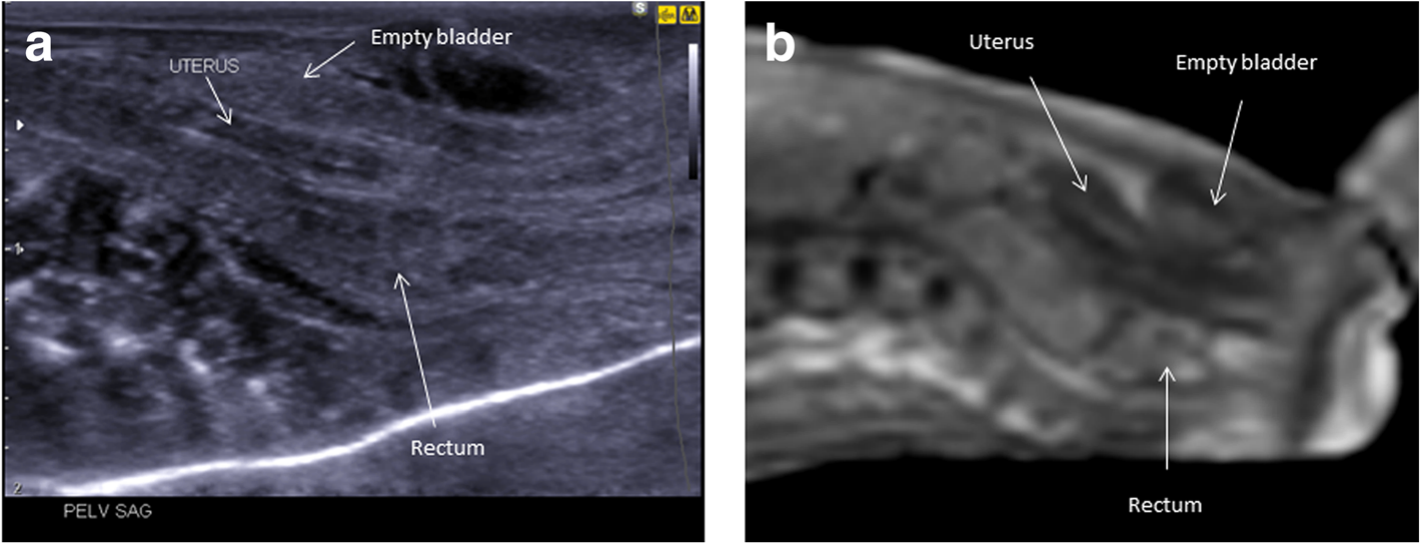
These images demonstrate normal appearances of the pelvic structures in a female 18-week gestational-aged foetus. Sagittal postmortem ultrasound of the pelvis (a) and corresponding postmortem T2-weighted MRI at 1.5 T (b) have been obtained 12 days after death. The tubular appearances of a normal pre-pubertal uterus, rectum and urinary bladder are all well seen and labelled in these images. Using a high-frequency linear probe, the pelvic anatomy can be better demonstrated on ultrasound rather than MRI
